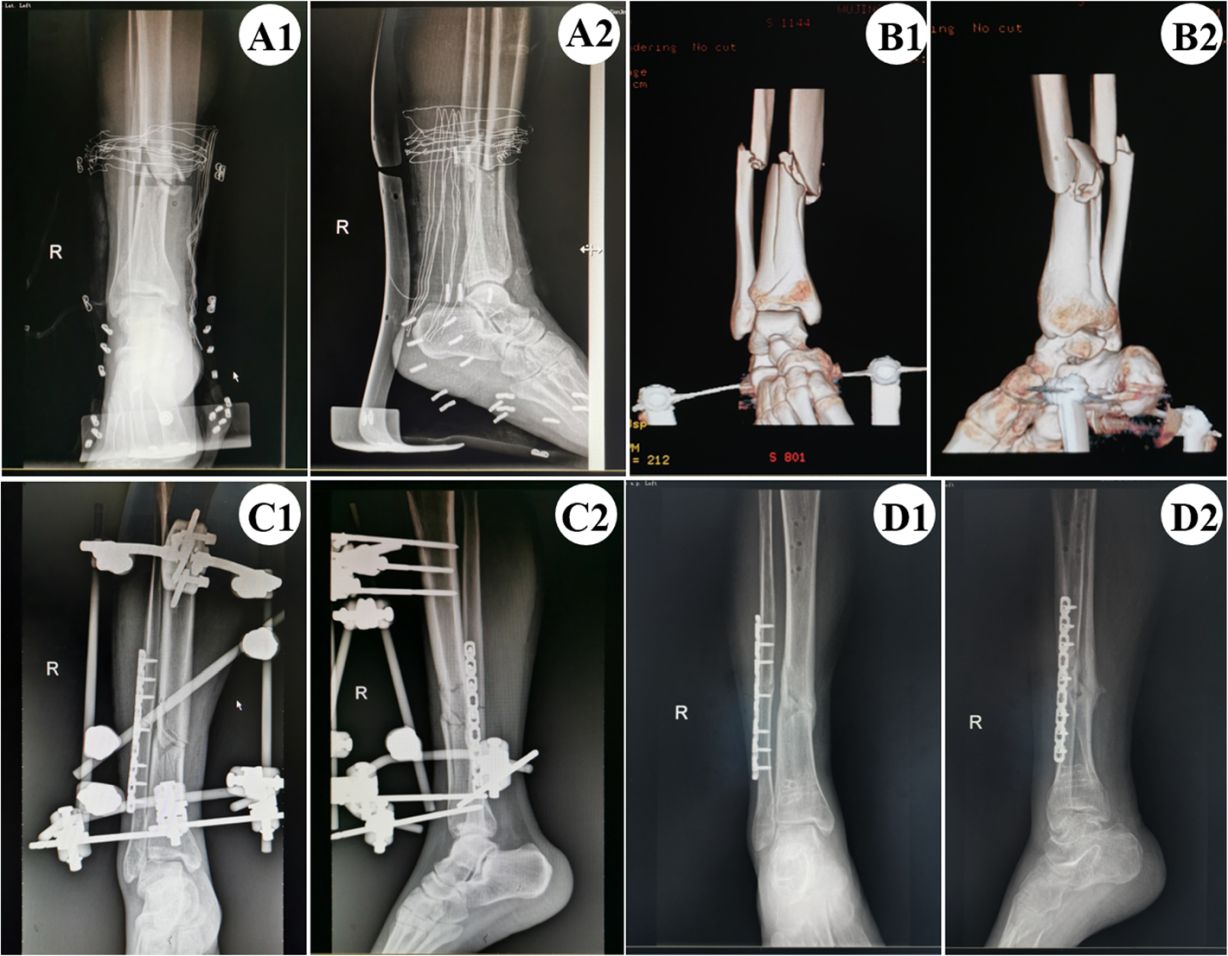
A1-A2 Anteroposterior and lateral X-ray examination of distal tibiofibular fractures before operation. B1-B2 The 3D reconstruction image of CT scan after calcaneal traction. C1-C2 Bedside X-ray radiograph 2 days after operation (anteroposterior and lateral views). D1-D2 The fracture showed radiological signs of healing 6 months after operation and the external fixator was removed completely (anteroposterior and lateral views)
